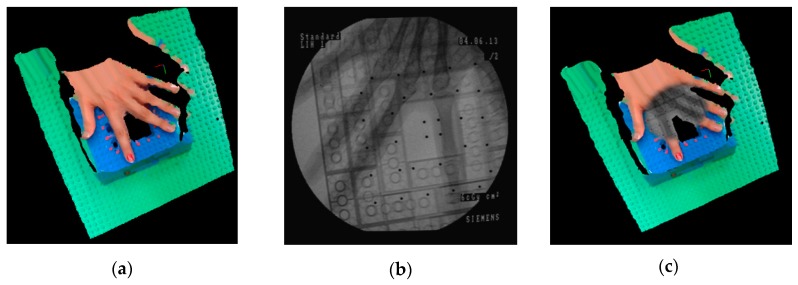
(a) 3D reconstructed model of the scene; (b) The corresponding X-ray image; (c) Visualization of the X-ray image on the 3D reconstruction of the scene by using texture mapping.
